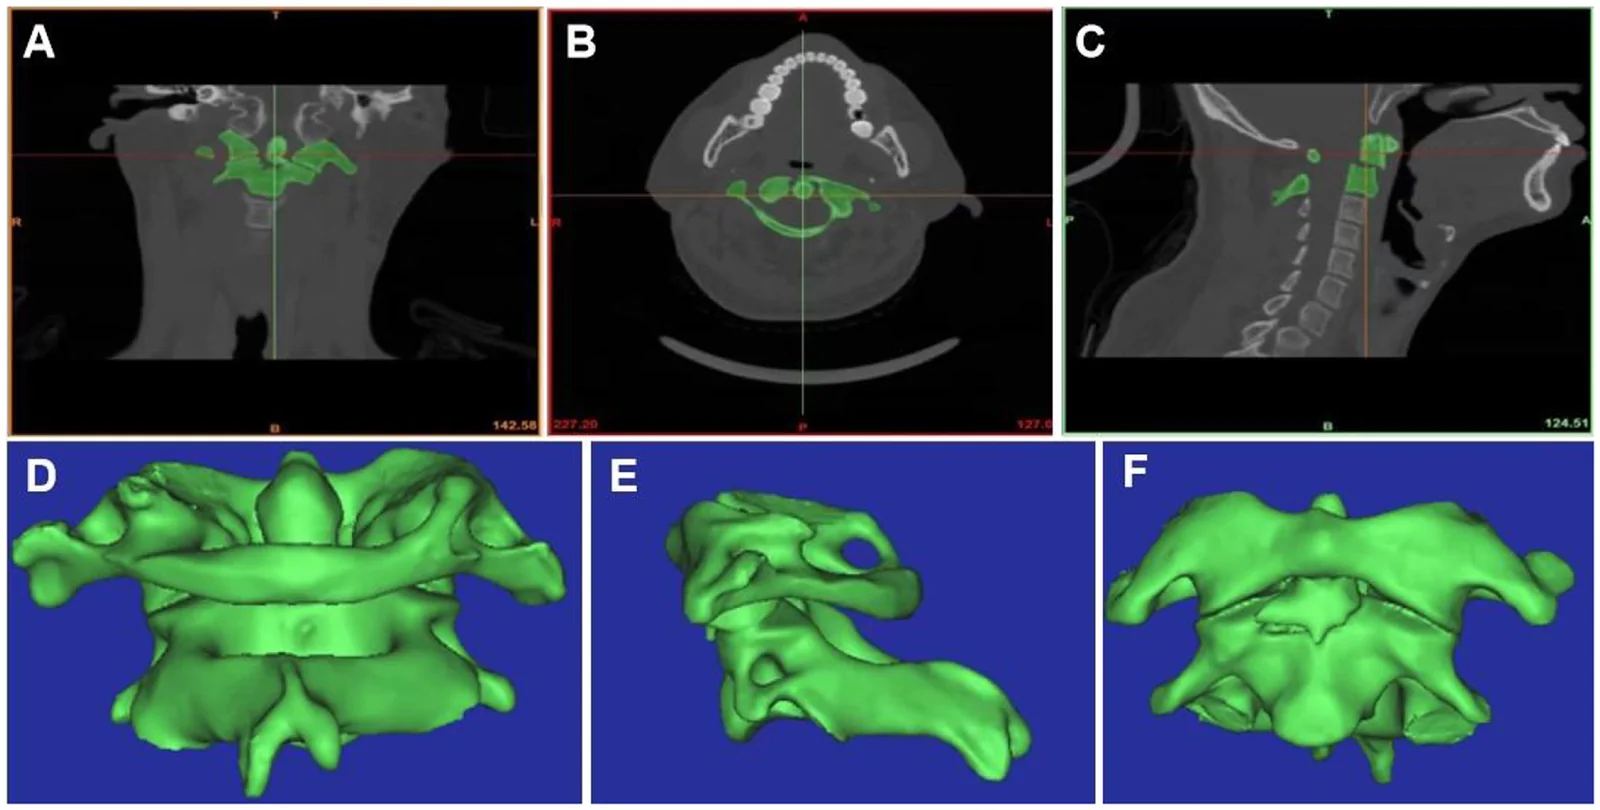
(A-C) CT images of the atlantoaxial vertebrae in the coronal, axial, and sagittal planes; (D-F) 3D reconstruction model of the atlantoaxial joint.
